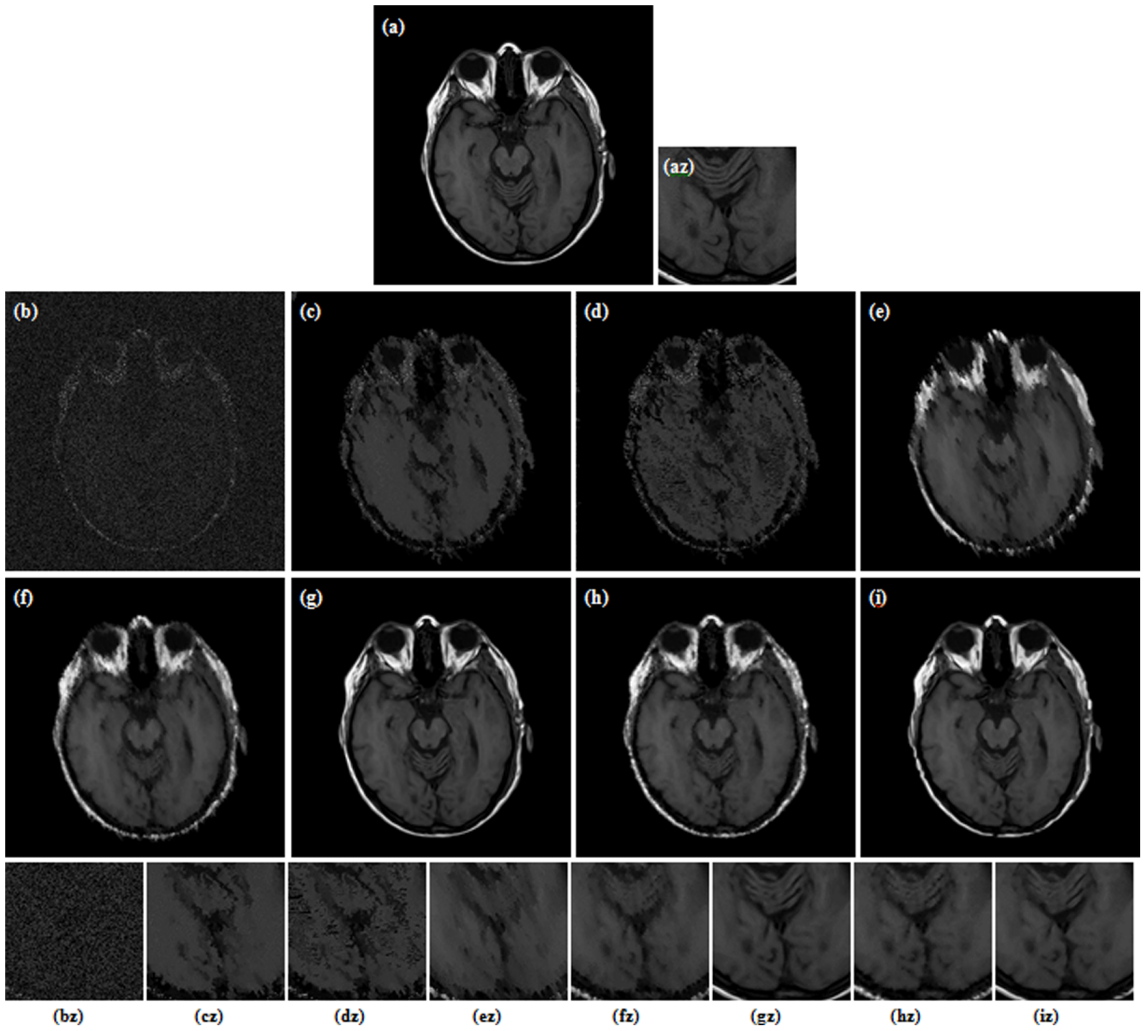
Restoration results for MRI image (90% salt-and-pepper noise). (a) Original image (b) Corrupted image (2.03 dB, SSIM = 0.0096). (c) AMF (6.91 dB, SSIM = 0.6051) [6]. (d) DBA filter (5.91 dB, SSIM = 0.5614) [7]. (e) MDBUTMF filter (9.30 dB, SSIM = 0.6623) [8]. (f) MDBUTAF filter (12.87 dB, SSIM = 0.7532). (g) SKR method (19.40 dB, SSIM = 0.8706) [23]. (h) RGMLE-C algorithm (16.81 dB, SSIM = 0.8426). (i) RGMLE-CS algorithm (18.92 dB, SSIM = 0.8717). (az)–(iz) denote the zoomed local parts for the corresponding images in (a)–(i).
